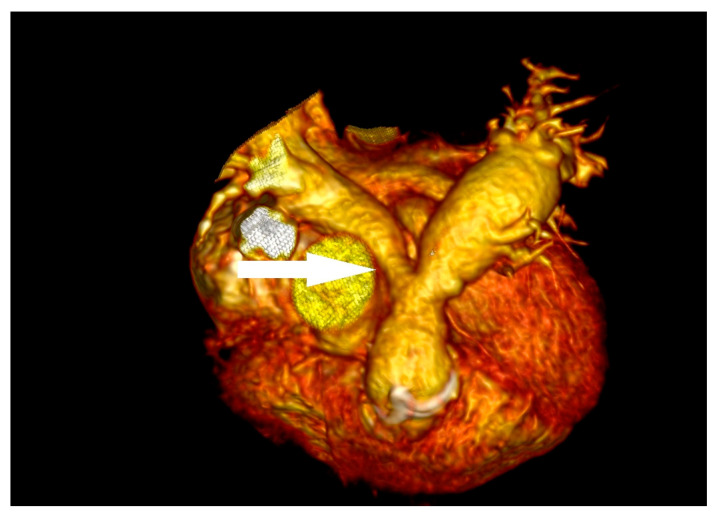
Three-dimensional (3D) model based on MR imaging; the arrow points at mild RPA stenosis; RPA—right pulmonary artery.
